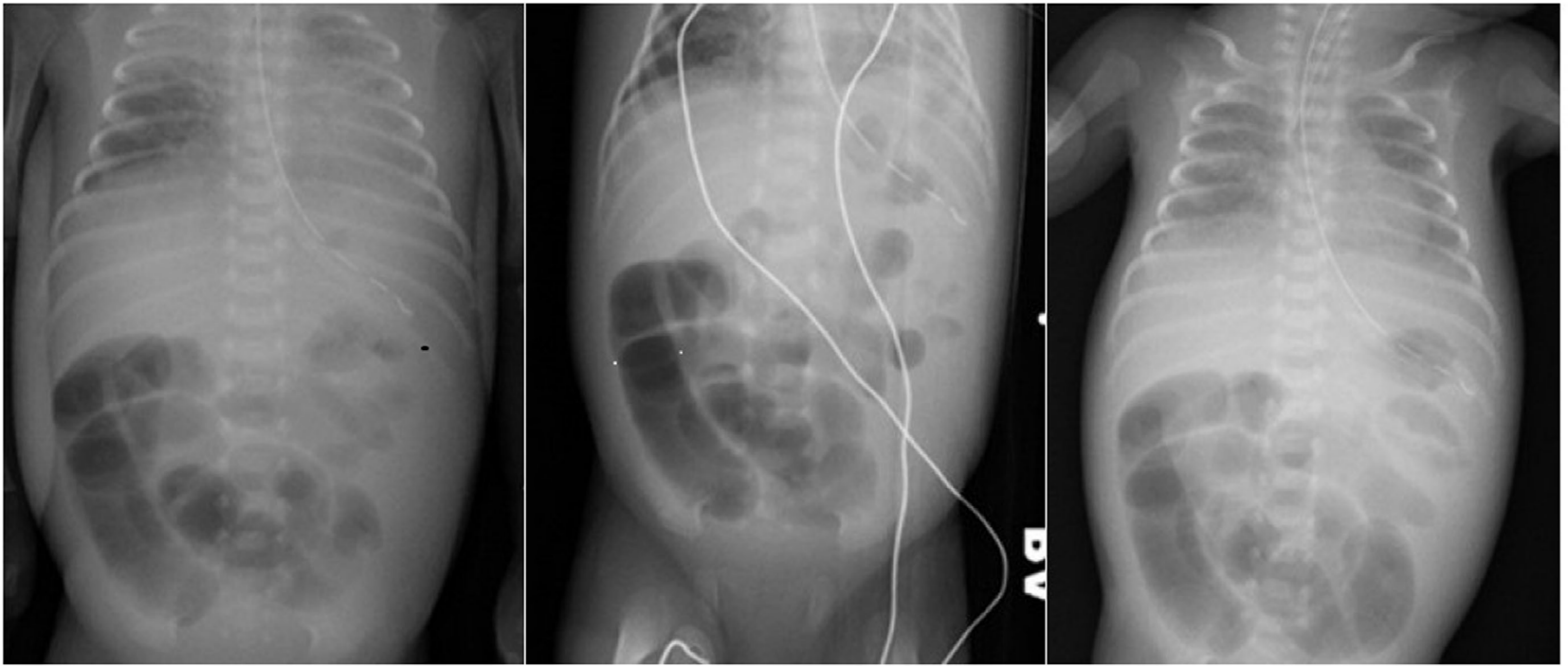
Left pane—plain AP radiograph of chest and abdomen with dilated loops of bowel. Central pane—plain left lateral decubitus radiograph of abdomen with dilated bowel loops and no evidence of pneumoperitoneum. Right pane—sequential AP radiograph of abdomen showing fixed dilated “sentinel” loops.
